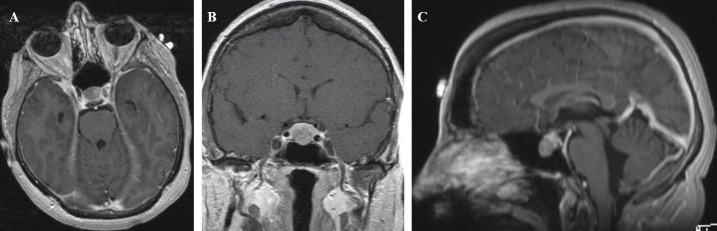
Preoperative T1-weighted MRI brain with and without gadolinium in the axial (A), coronal (B), and sagittal (C) planes showing a 11.5 x 14.0 x 10.0 mm hypoenhancing mass in the inferior pituitary gland causing upward bowing of the diaphragma sella and mild sellar expansion.
